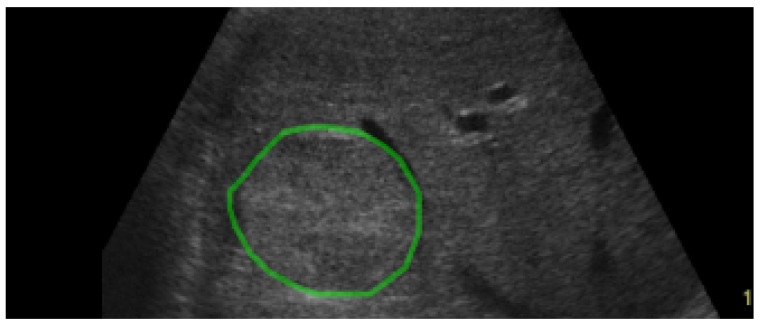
The visual aspect of HCC in ultrasound images: the HCC contour is marked with green.
